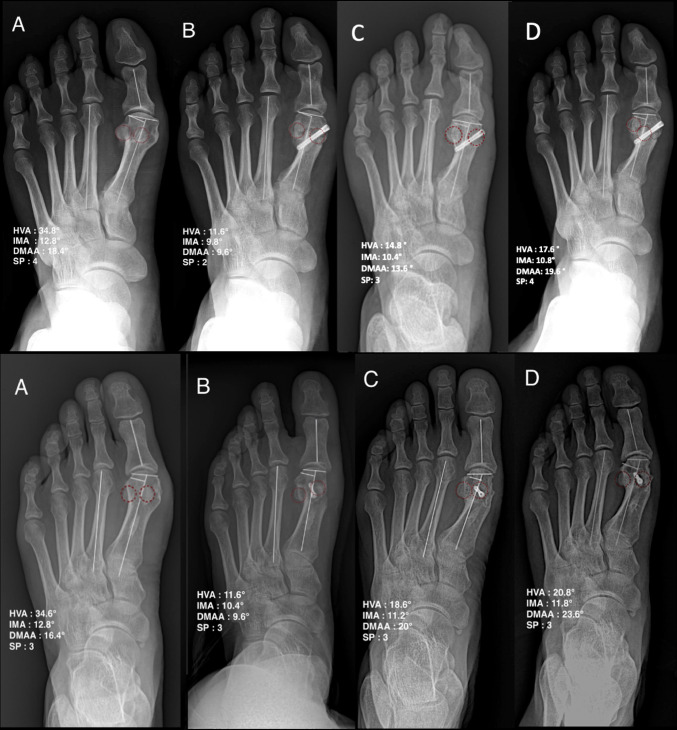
Baseline-matched weight-bearing AP radiographs (recurrent; final HVA > 15°), same layout/time points as Fig. 4. Overall recurrence: 4.3% (Modified) vs 27.8% (Chevron). Red circles indicate the sesamoid complex.
